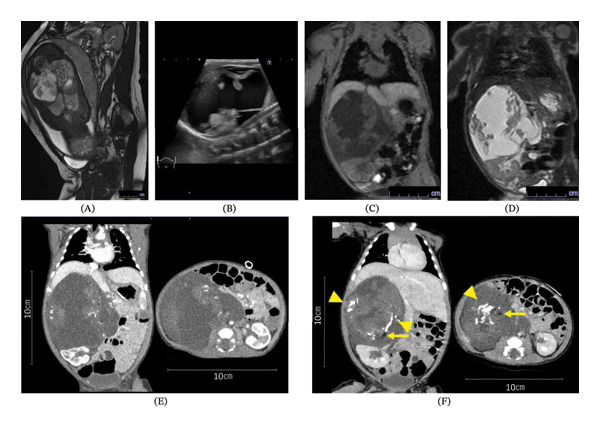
Imaging findings. (A) Fetal MRI at 36 weeks’ gestation demonstrates a 58‐mm cystic–solid mass located between the right kidney and liver. The lesion is predominantly cystic with a small solid component. (B) Postnatal ultrasonography on Day 5 shows an 80‐mm cystic–solid mass in the same region; the right adrenal gland is not clearly identified. (C) Postnatal T1‐weighted MRI on Day 5 reveals a 77‐mm cystic–solid mass at the upper pole of the right kidney; the solid component is isointense. (D) Postnatal T2‐weighted MRI on Day 5 shows that the solid component is hypointense. (E) Contrast‐enhanced CT on Day 7 demonstrates an 80 × 78‐mm cystic–solid mass with coarse calcifications; the right adrenal gland is not identified. (F) CT on Day 23 shows newly apparent fatty components (arrow) and linear calcifications (arrowhead) within the mass, which measures 84 mm in maximum diameter.
